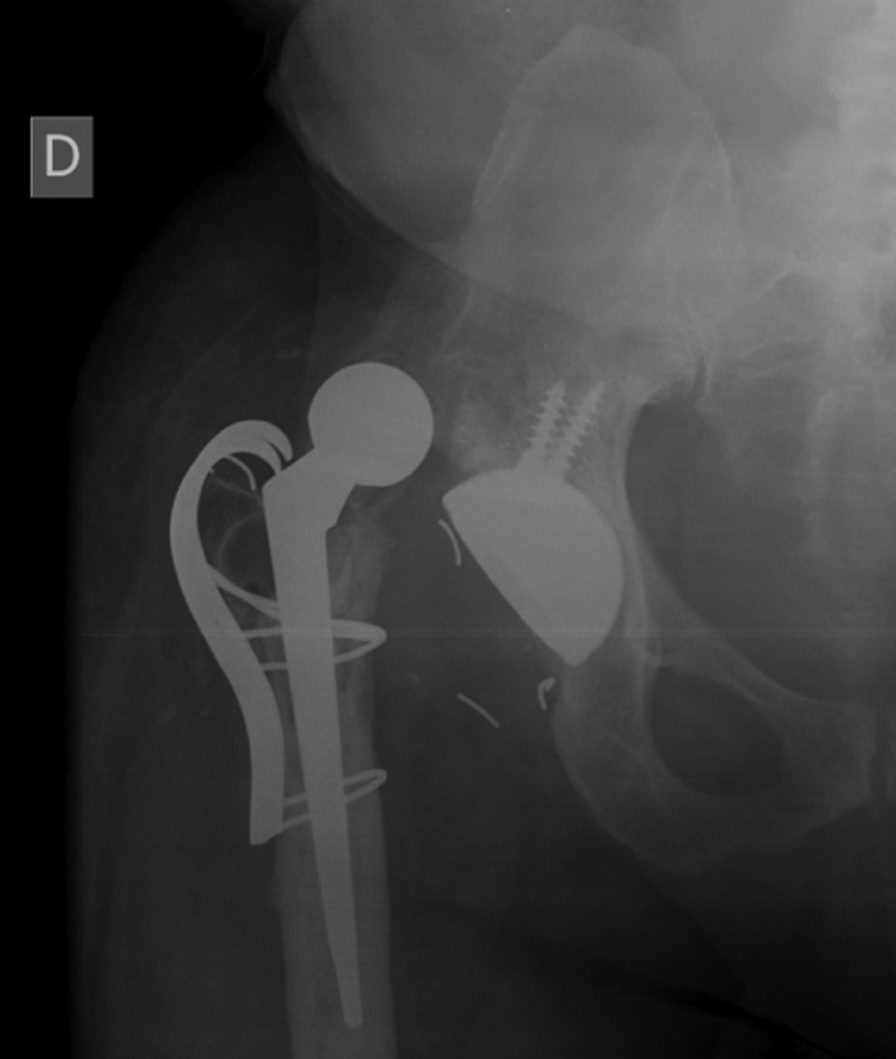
Anteroposterior hip radiograph showing prosthetic hip dislocation.
